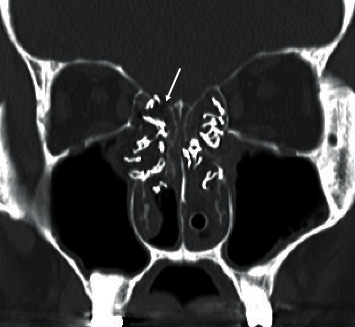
The head and neck computed tomography (CT) radiological findings. The head and neck coronal CT in the bone window setting revealed a defect in the cribriform plate (arrow), consistent with a cerebrospinal fluid leak.
